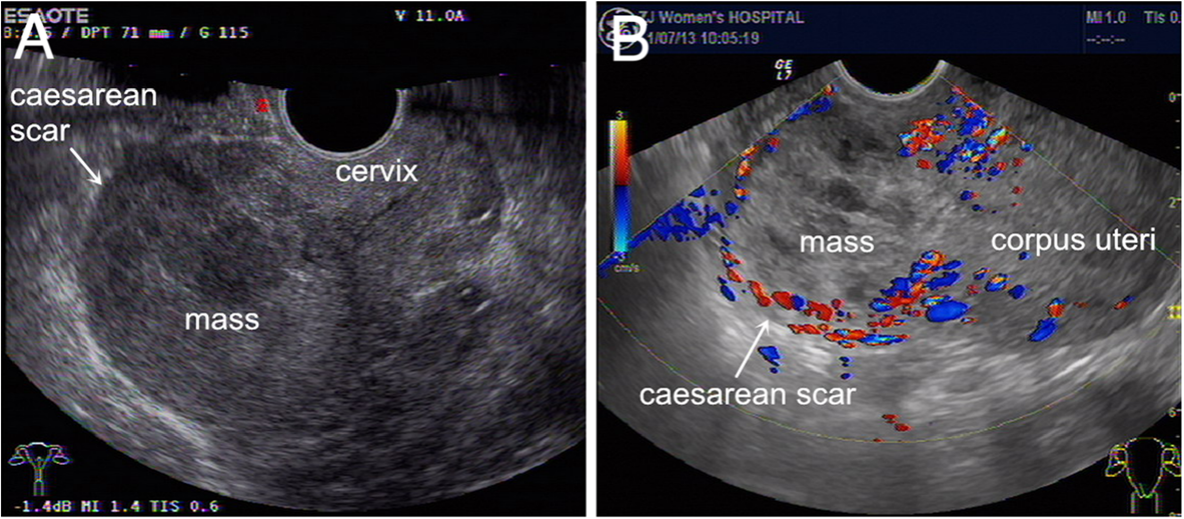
Transvaginal ultrasonography of the patient before (A) and after (B) dilation and curettage (D&C). The uterine cavity and cervical canal were empty. A mass implanted in the anterior wall of the uterine Caesarean scar (indicated by the arrow) embedded and surrounded by thin myometrium and separated from the endometrial cavity was visible on the retroverted uterus. Ultrasonography revealed that the mass was heterogeneous with a mixture of cystic and solid echogenicity. (A) Two days before D&C (longitudinal section). The size of the mass was 6.6 × 5.6 × 5.5 cm. RI = 0.38. (B) Five days after D&C (longitudinal section). The size of the mass was 4.7 × 5.7 × 4.5 cm. Abundant blood flow signals and low RI around the mass, RI = 0.29.
